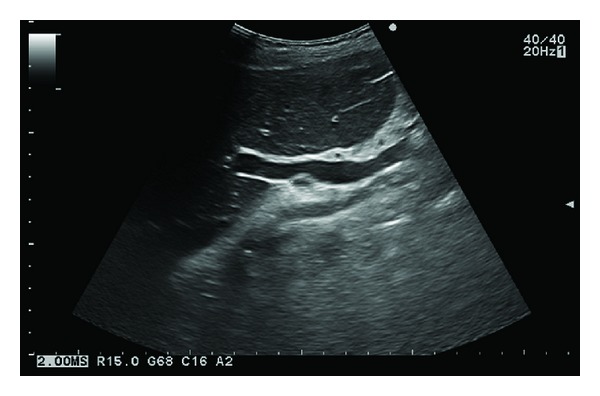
Ultrasonography with the typical technique and the probe placed at the right hypochondrium could not locate the gallbladder at the plane of the liver hilum.
